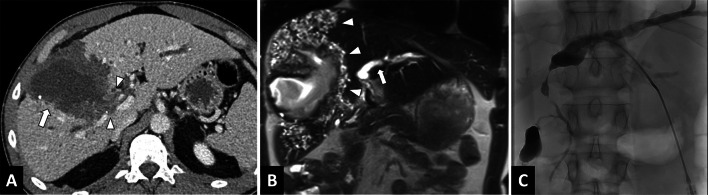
Large alveolar echinococcosis lesion of the right liver in a 34-year-old man diagnosed with jaundice. A CT image in the axial plane obtained at portal phase of enhancement showing alveolar echinococcosis of the right liver (arrow) associated with infiltration up to the right pedicle (arrowheads) and the biliary convergence. B Coronal T2-weighted MR images. Multiple microcysts (arrowheads) with biliary convergence involvement leading to biliary dilation in the left lobe (arrow). C Cholangiography images. Percutaneous biliary drainage to achieve regression of jaundice in preparation for liver surgery
